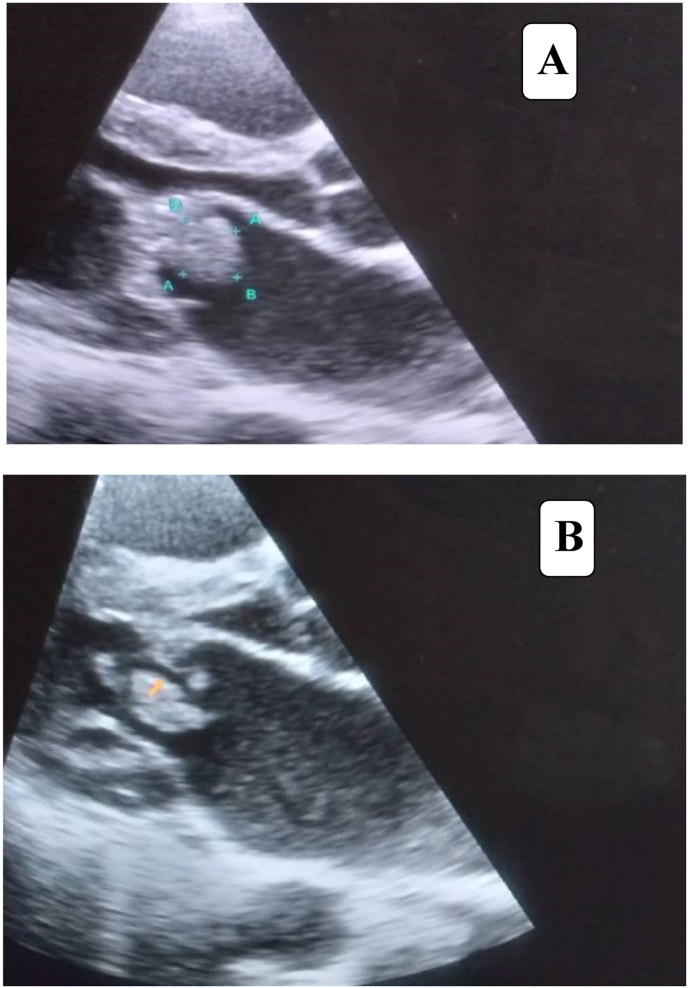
(A&B): Transthoratic echocardiography shows an oscillating mass 16mm*6mm attached to the anterior of mitral valve (typical of a vegetation) and letf atrium dilatation 42MM (A). Typical destructive vegetation with clear prolapse of the mass in to left atrium (B).
